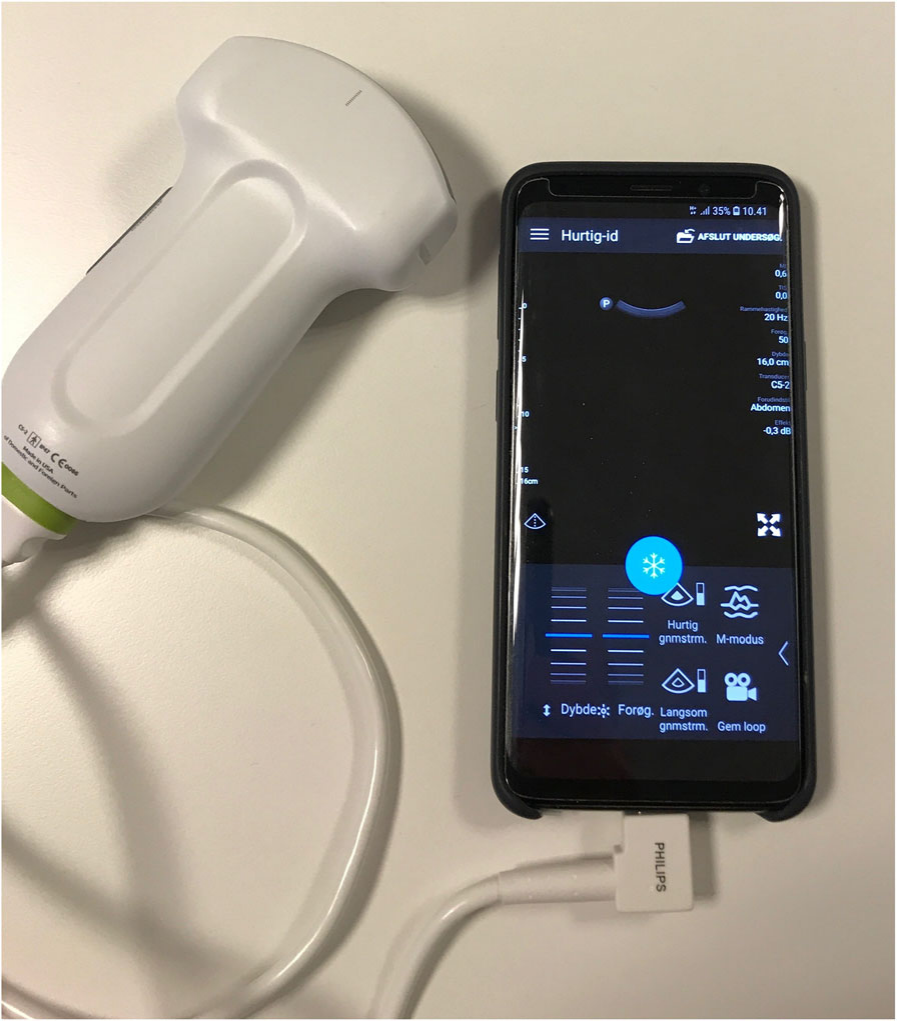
Lumify portable ultrasound device
